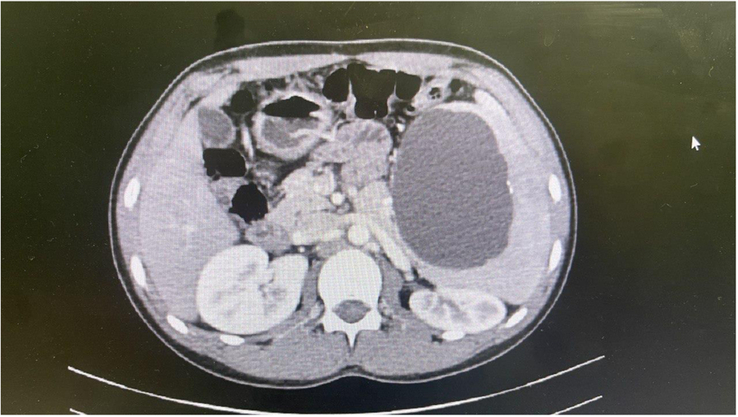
A computed tomography affirms the diagnosis of the giant splenic hydatid cyst. Several calcified foci are seen in the cyst wall. The germinal layer is intact and there is no sign of cyst rupture is visible. There are no signs of other organ involvement, most importantly liver and lung.
